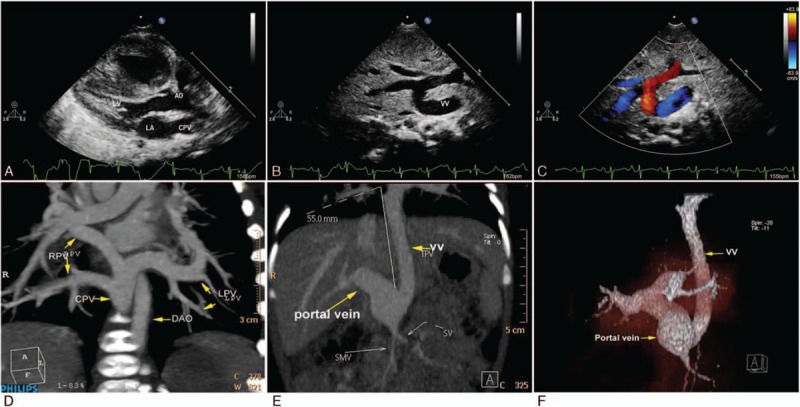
Echocardiography and computerized tomography angiography (CTA) show the infracardiac total anomalous pulmonary venous connection of a newborn baby. (A) The parasternal long-axis view shows the common pulmonary vein (CPV) in the lateral side of the left atrium. (B) The subcostal view shows the descending vertical vein (VV) draining to the portal vein. (C) Color Doppler shows the flow from VV to portal vein. (D) CTA displays the 4 pulmonary veins confluence drains into the descending VV (DVV). (E) CTA shows how the CPV traverses the diaphragm. (F) Computerized tomography volume rendering shows the DVV draining into the portal vein.
